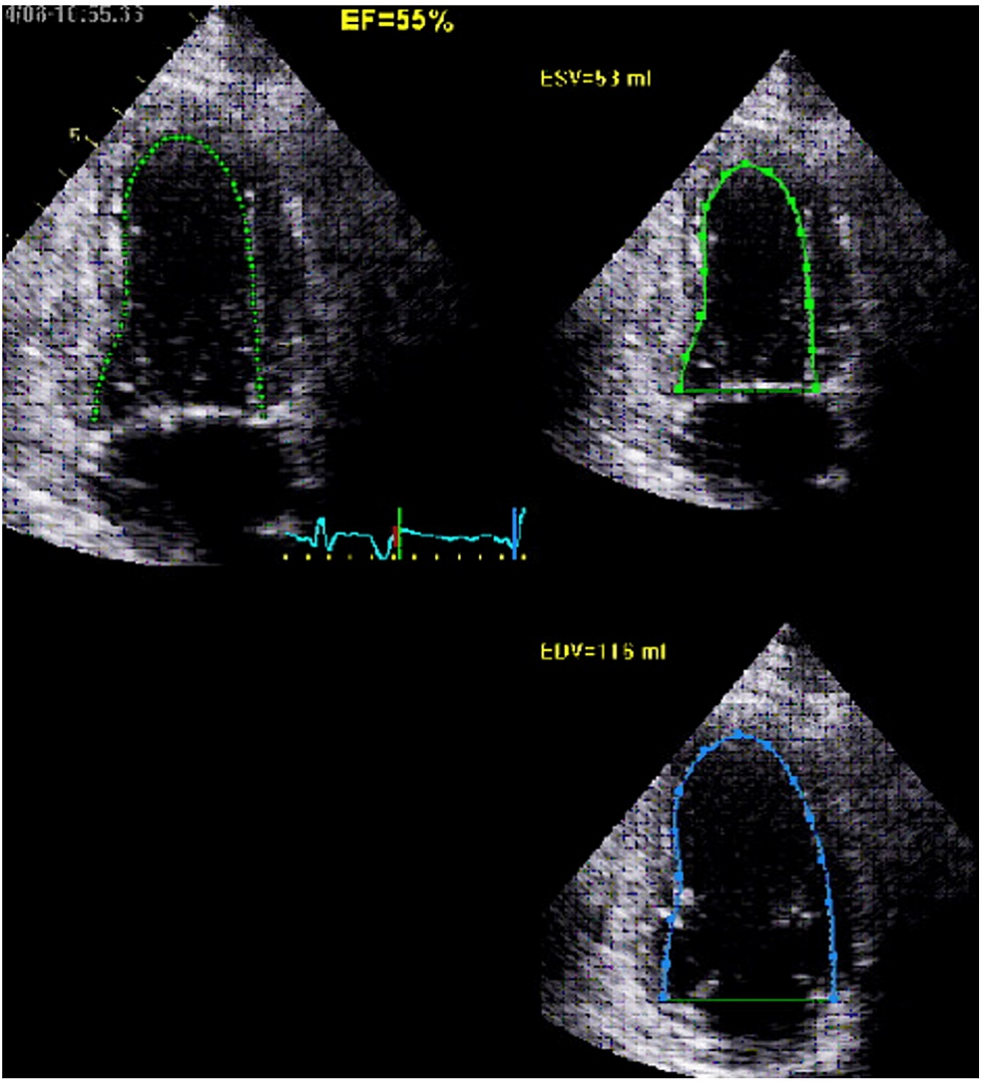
Three-month echocardiogram showing normal left ventricular chamber size, mildly decreased left ventricular ejection fraction estimated at 40-49%, with akinetic apex, and hypokinetic inferior wall and anteroseptal wall
